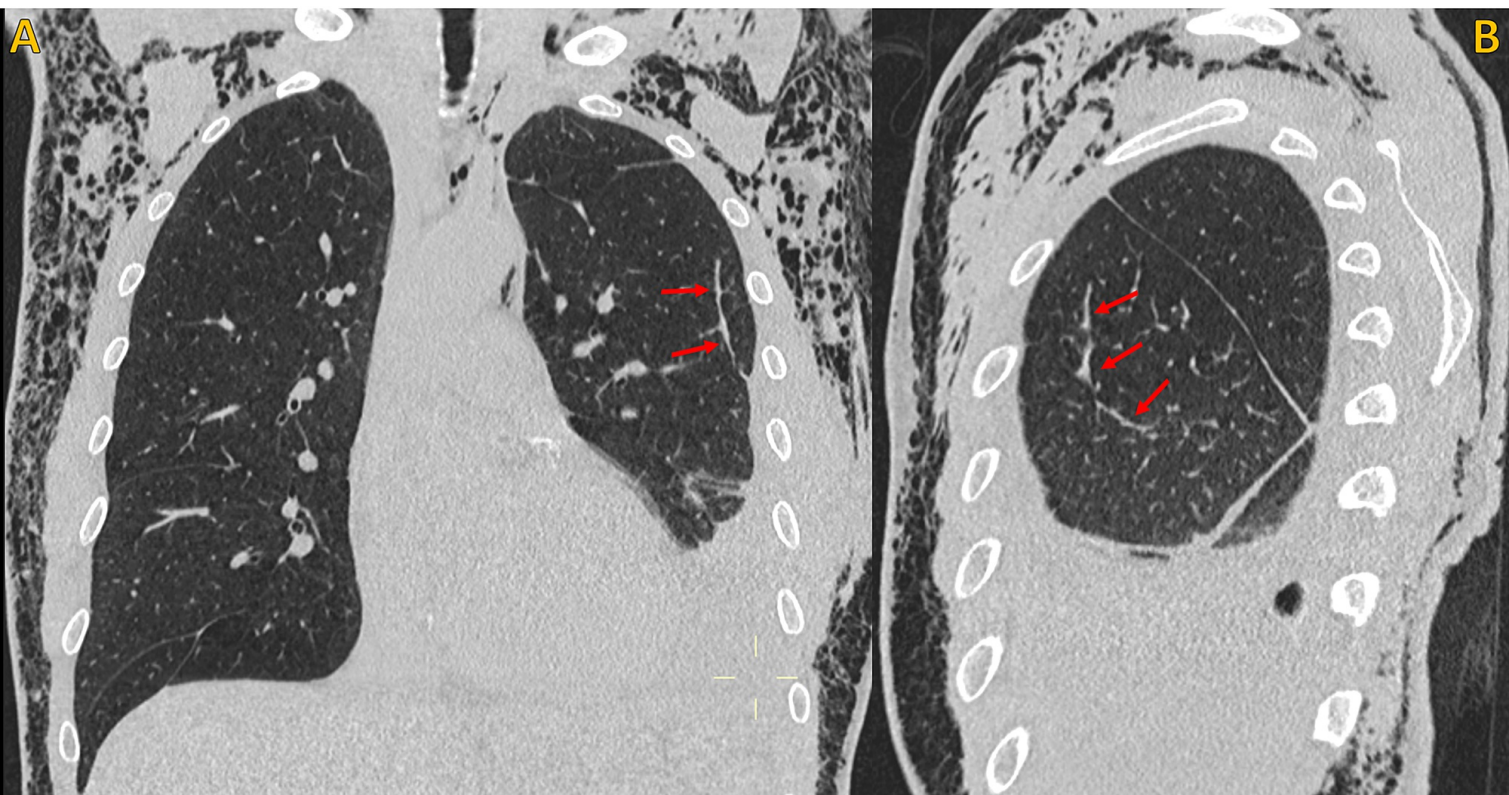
Non-contrast chest CT, lung window Non-contrast chest CT, lung window. A) Oblique coronal plane and B) Oblique sagittal plane showing that there is no longer evidence of the peripheral enlarged bronchiolar structure on the left lung neither the point of its communication with the pleural space. In its location, there is now an elongated and filiform structure, with soft tissue density and a fibrous and slightly retractable aspect, sequelae findings derived from the closure of the bronchopleural fistula (red arrows).
